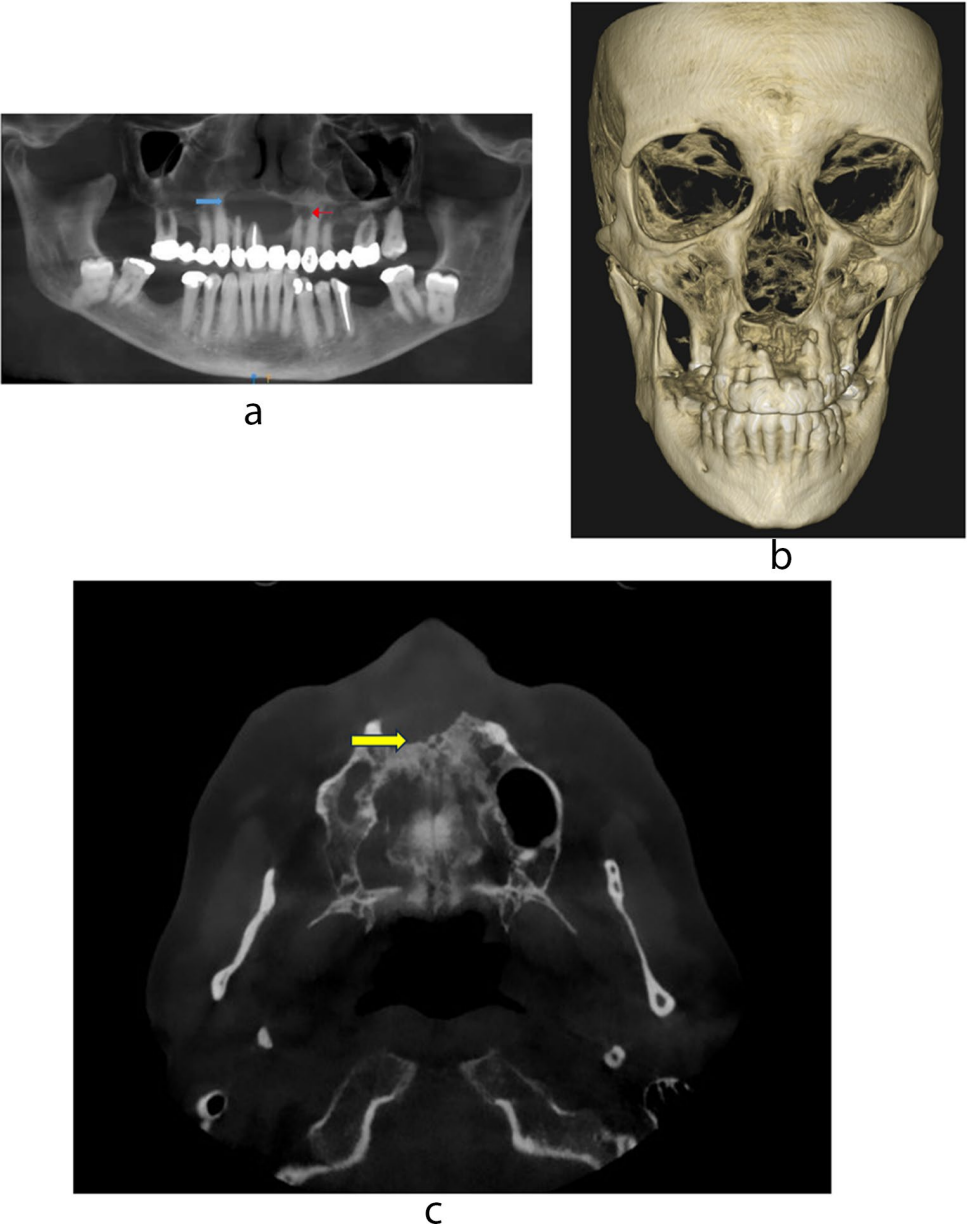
a CBCT panoramic reconstruction shows a large well circumscribed multilocular radiolucency associated with the apices of teeth 10,9 and 7. Root canal treated tooth 9 suggests a periapical cyst (blue arrow larger locule and red arrow smaller locule). b A 3D MIP shows destruction of the anterior maxillary bone. c CBCT scan shows the posterior border of the lesion with cortical bone destruction and narrow transition zone involving nasopalatine foramen (yellow arrow)
